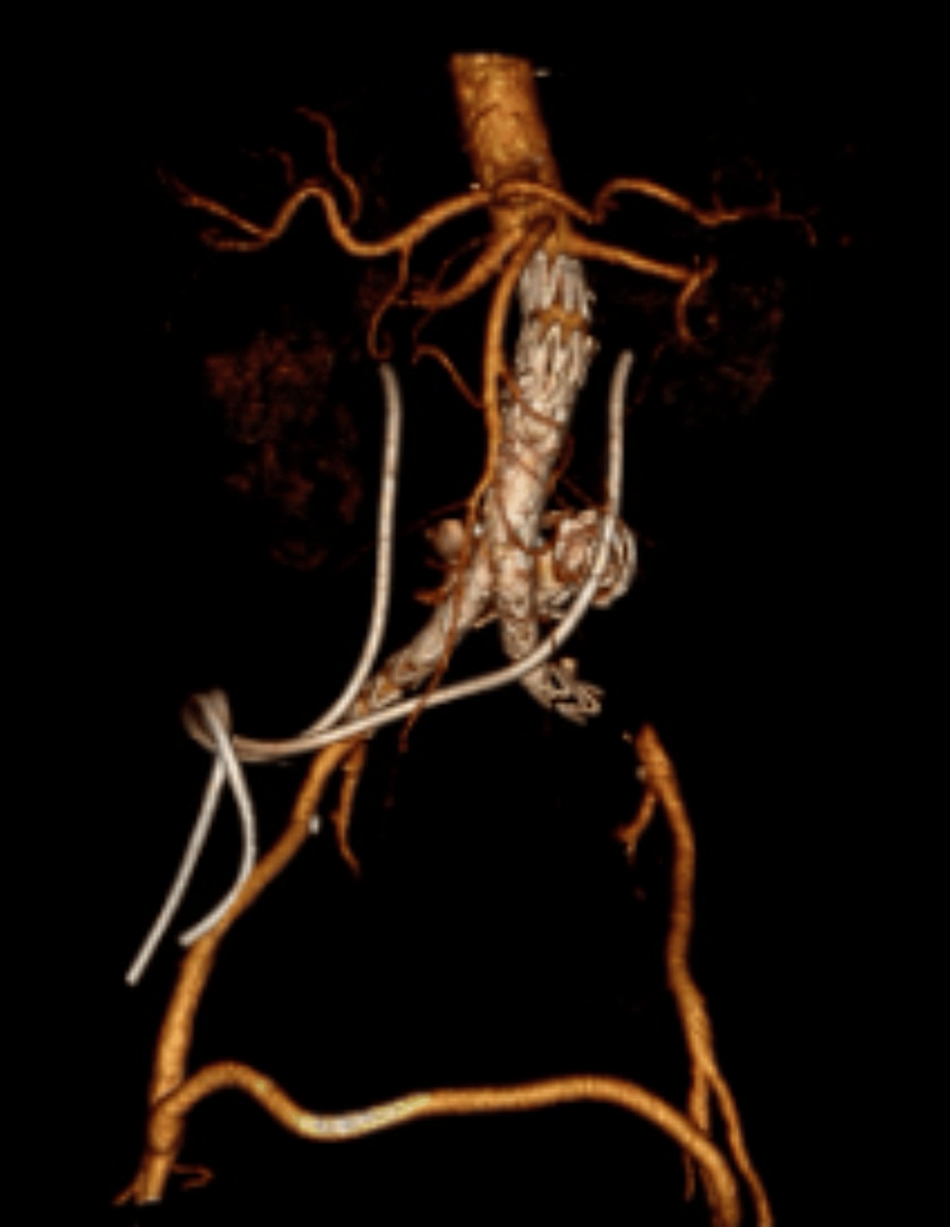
Post-procedural CTA showing the final result. CTA: computed tomography angiography
